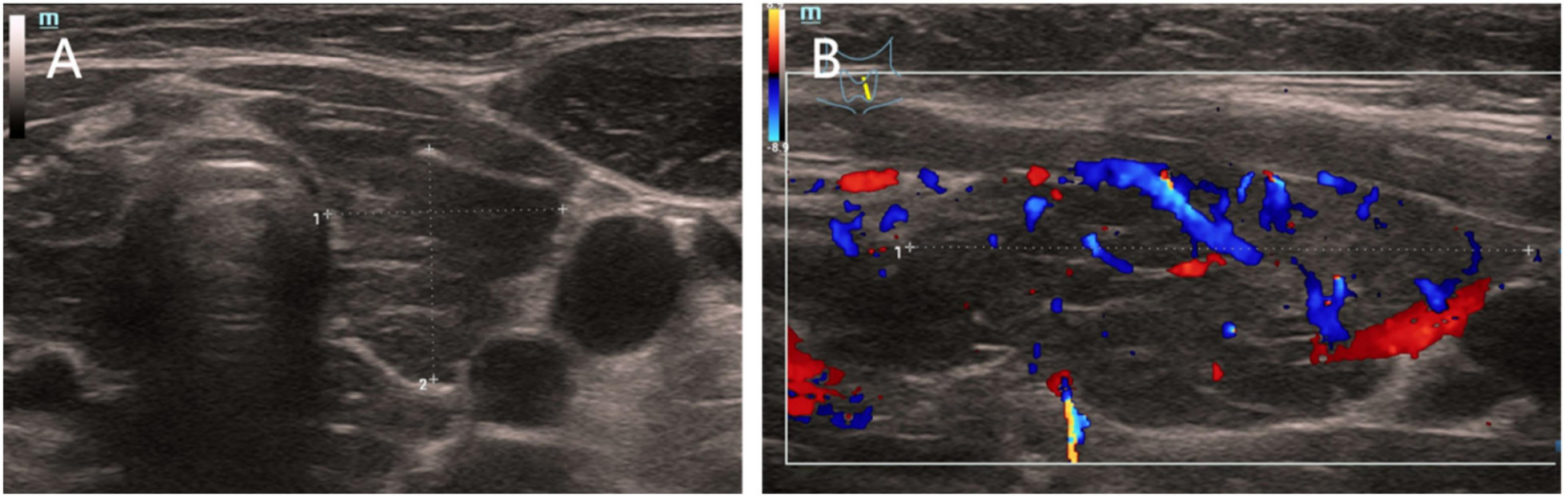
Thyroid color Doppler ultrasound. (A) Diffuse enlargement of the right thyroid lobe with coarse echotexture.The right thyroid lobe measured 1.39 cm × 1.36 cm. (B) Increased intraglandular vascularity consistent with Hashimoto's thyroiditis (C-TIRADS Class 1).
